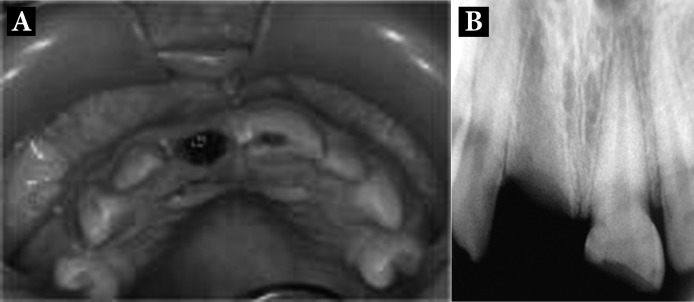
A) Intraoral examination; B) Radiographic examination
